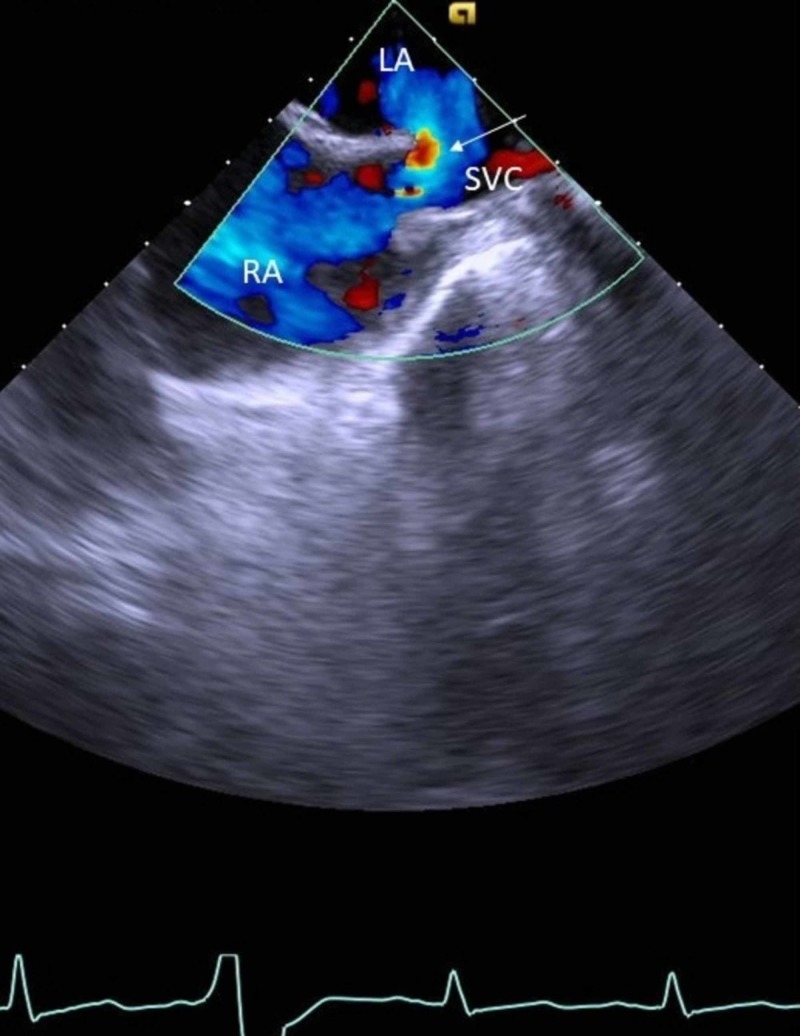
Transesophageal echocardiography with Doppler The arrow shows left-to-right shunting. LA, left atrium; SVC, superior vena cava; RA, right atrium
